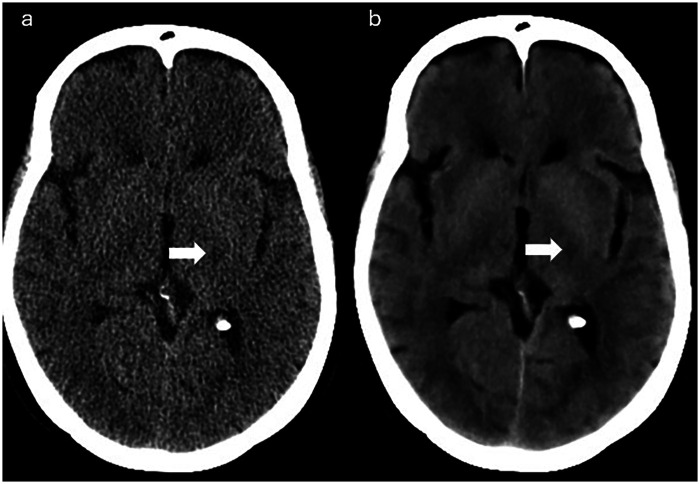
Axial CT LD images of a 36-year-old woman with a left chronic infarction. The small hypoattenuation area (arrows) was clearly delineated in DLIR (b) while preserving image noise compared with iDose^4^
